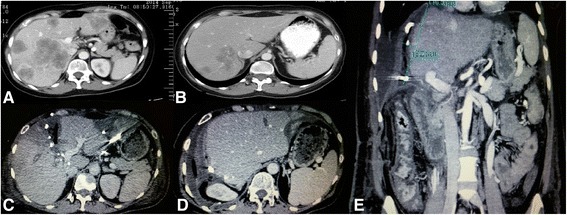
a Pre-chemotherapy abdominal CT scan showing multiple liver mestastases. b Post-chemotherapy abdominal CT scan showing response to treatment. c, e Abdominal CT scans showing adequate volume expansion after the step 1 of ALPPS procedure. d Postoperative abdominal CT scan without evidence of disease recurrence
